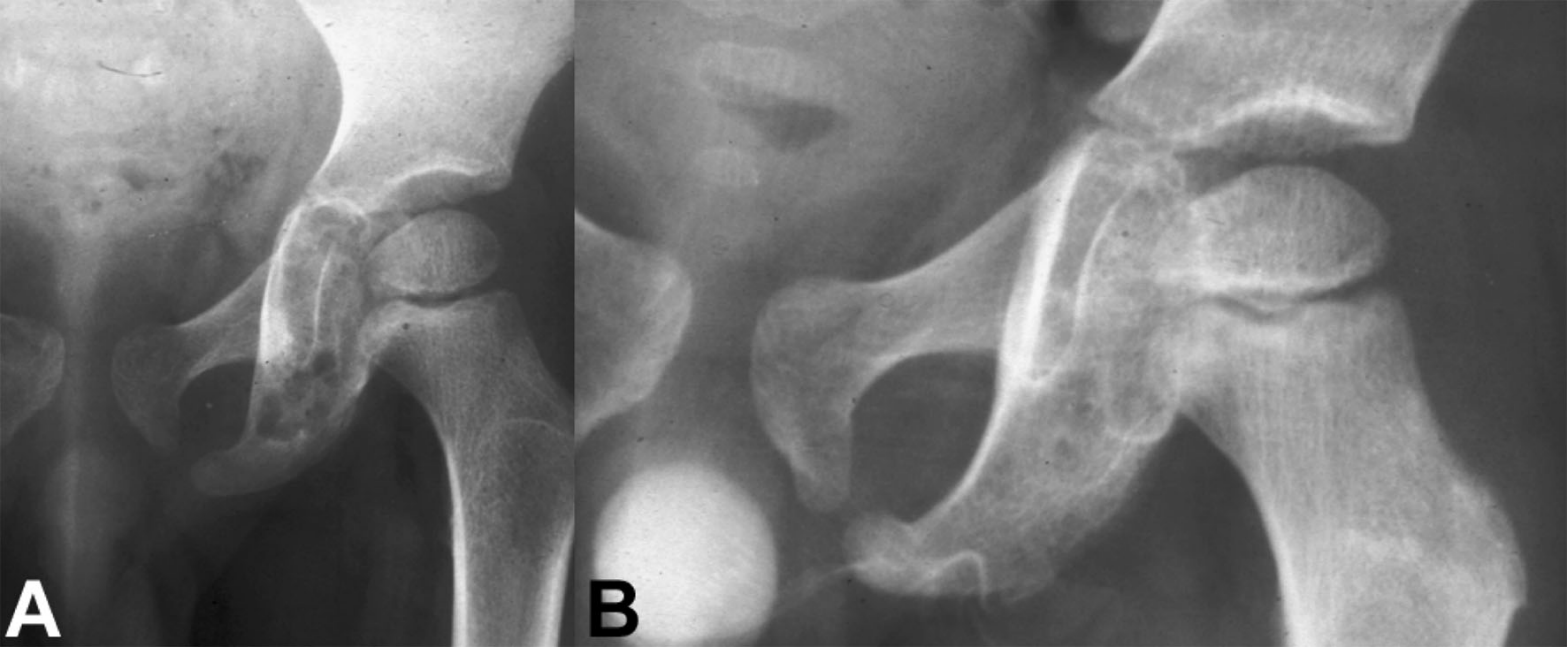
a Anteroposterior radiograph of the pelvis of a 6-year-old boy with a painful osteolytic lesion at the left ischial ramus. CT-guided frozen section biopsy showed eosinophilic granuloma; intralesional methylprednisolone injection was performed. b Anteroposterior radiograph of the pelvis shows complete reconstitution of the lesion 1 year after diagnosis and treatment
